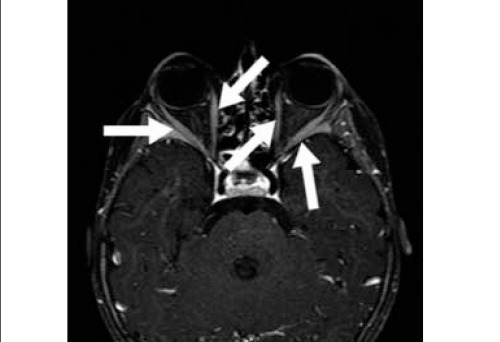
T1 weighted fat saturated contrast enhanced axial MR imageshows marked enhancement (arrows) of extraocular muscles of bilateral orbits
